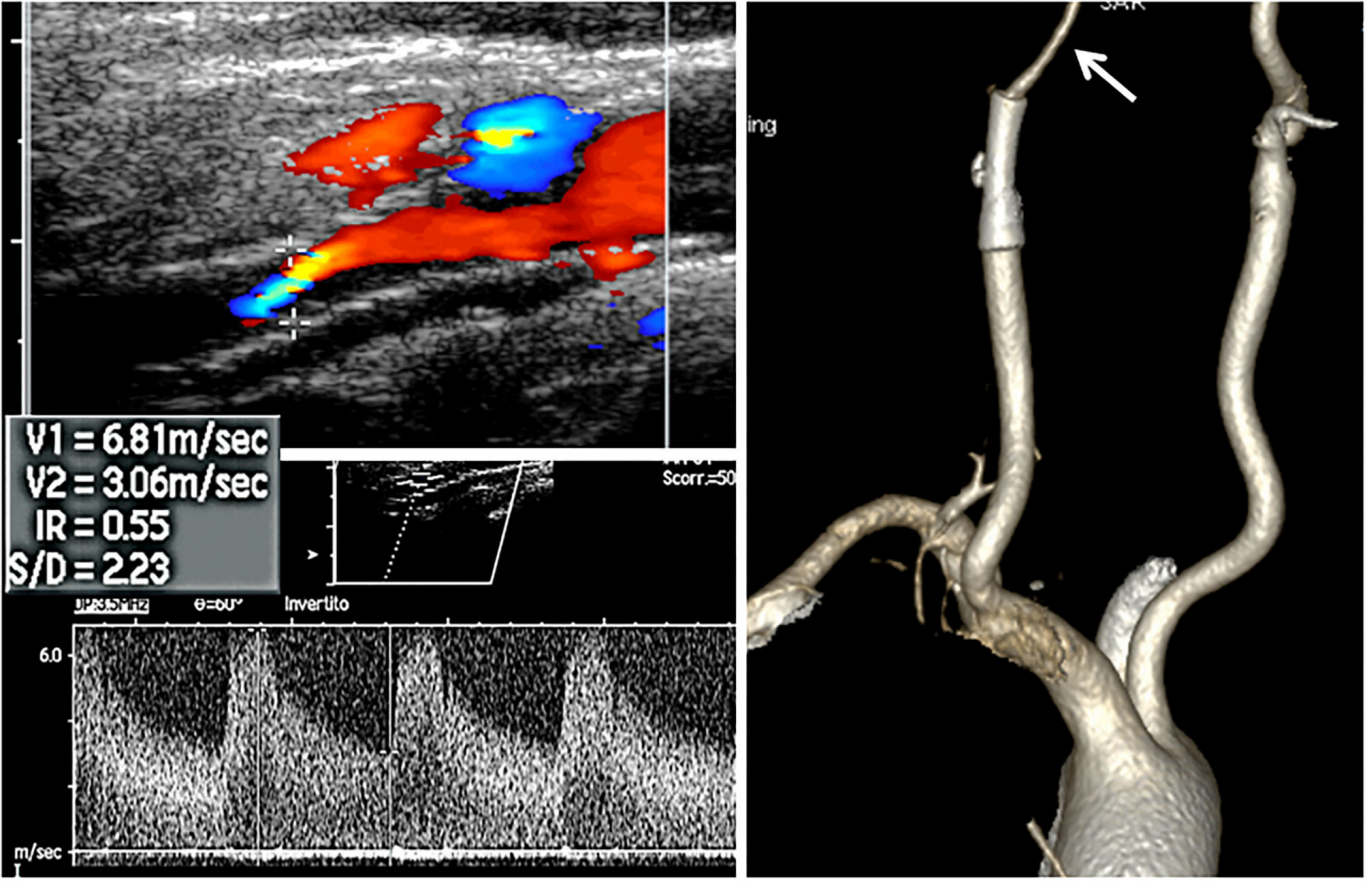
Non-invasive assessment. Doppler ultrasound (US, left side) and computed tomography (CT, right side) volume rendering CT angiography of the patient. Arrow indicates the lumen collapse distal to the stent.
